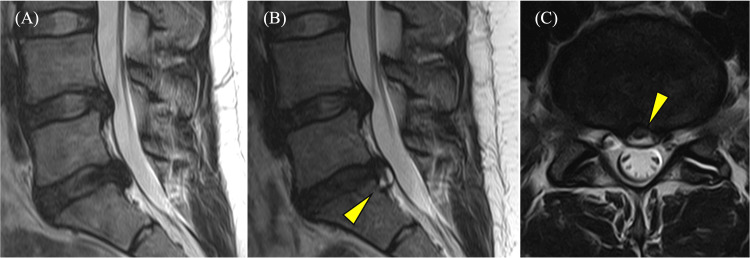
MRI before the first surgery. Sagittal T2-weighted MRI (A) three years prior showing bulging or lumbar disc herniation at the L4/L5 and L5/S1 levels. Sagittal (B) and axial (C) T2-weighted MRI taken before the first surgery showing no change in bulging at the L4/L5 level, worsening compression of the dural sac, and appearance of a high-intensity zone (arrowhead) at the L5/S1 level. MRI, magnetic resonance imaging
